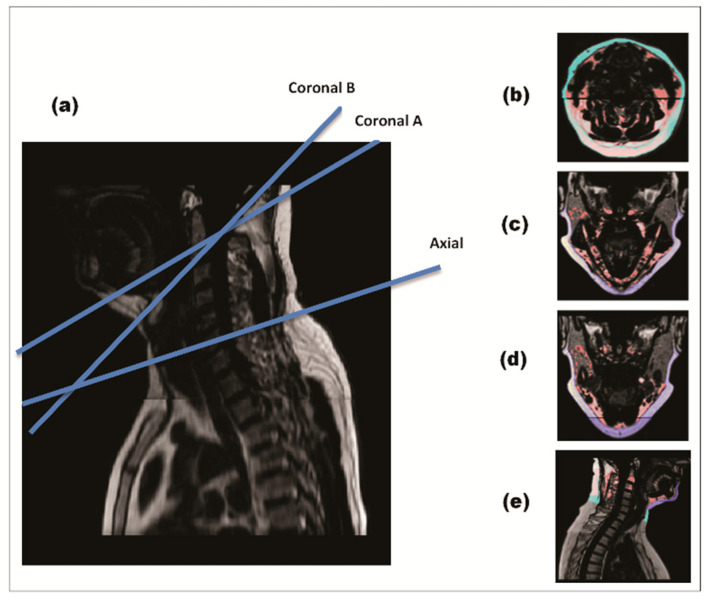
MRI of cervical-subcutaneous-adipose-tissue. Four anatomical slices per subject were extracted for each time point in each time. During quantification, cervical-SAT was divided into two sub-compartments—chin-SAT (dark blue) and cervical-SAT (light blue). Image (a) demonstrates a mid-sagittal section of the cervical region with blue lines placing the two coronal and axial sections. Image (b) demonstrates an axial slice, Image (c) coronal A slice, Image (d) coronal B slice and Image (e) sagittal slice.
